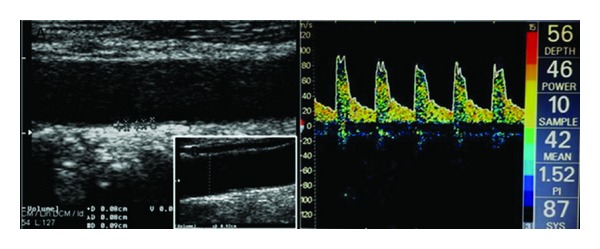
Ultrasound measurements. (A) CCA-IMT. (B) CCA diameter. (C) MCA-PI.
